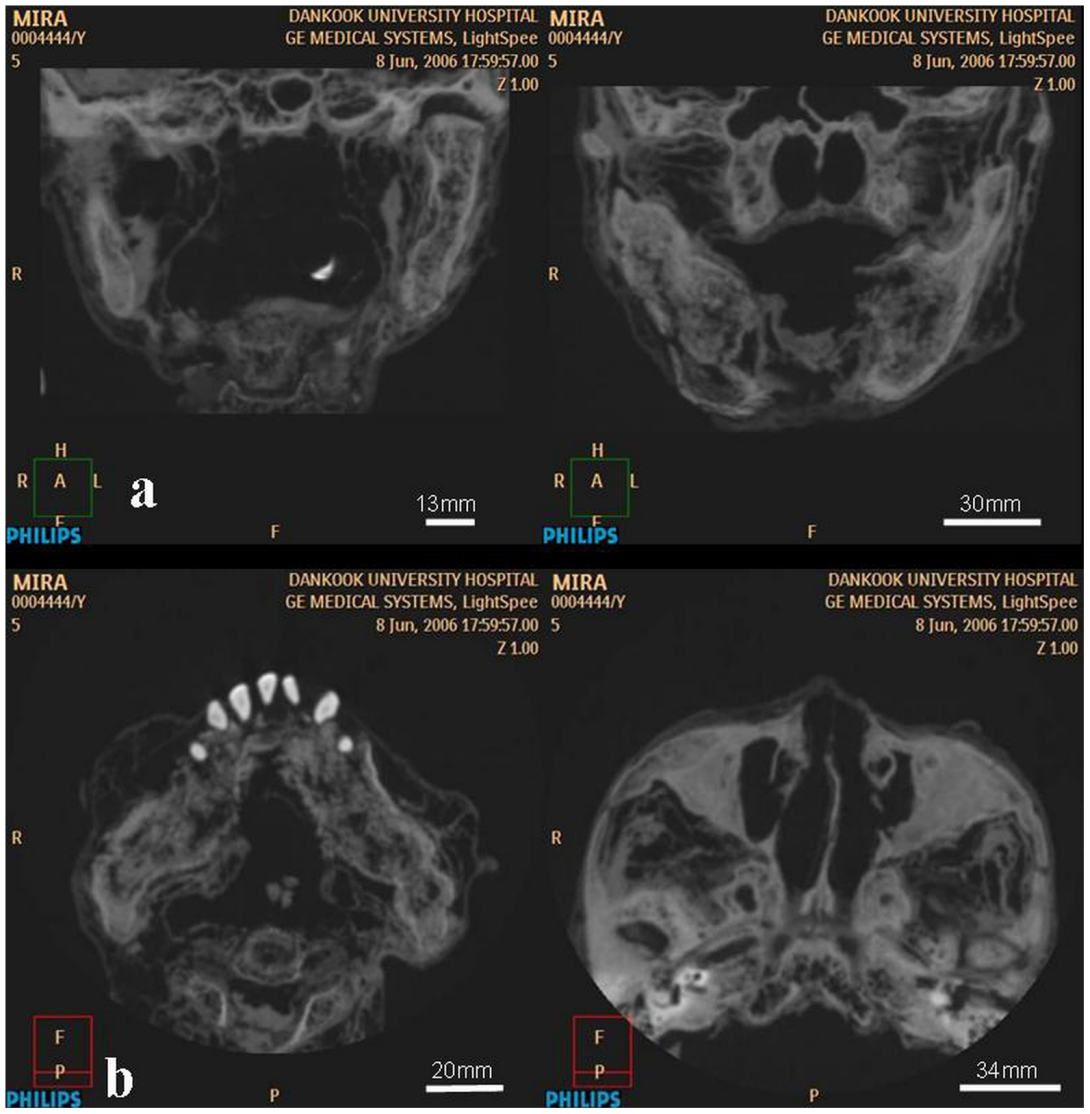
CT slices through the level of the maxilla and mandible. (a) Coronal images demonstrate symmetric, “bubbly” expansion of the maxilla (including orbital floor and maxillary buttresses) and mandible (with diffuse involvement of the body, ascending ramus and condyle). (b) Axial images demonstrate marked expansion of the mandibular body and ramus, disorganized spacing of the teeth (with missing molars), and thickening of the zygomaxillary area.
